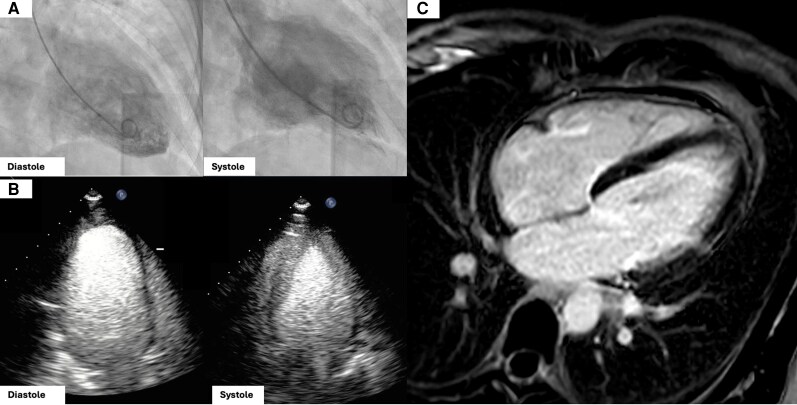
Initial cardiac imaging. (A) Ventriculography during diastole and systole with basal hypokinesis and conserved apical contraction. (B) Transthoracic echocardiogram in diastole and systole demonstrating anterolateral and inferolateral hypokinesis with preserved apical contraction. (C) Cardiac magnetic resonance demonstrating decreased resting perfusion in the subbasal endocardium and no late gadolinium enhancement.
